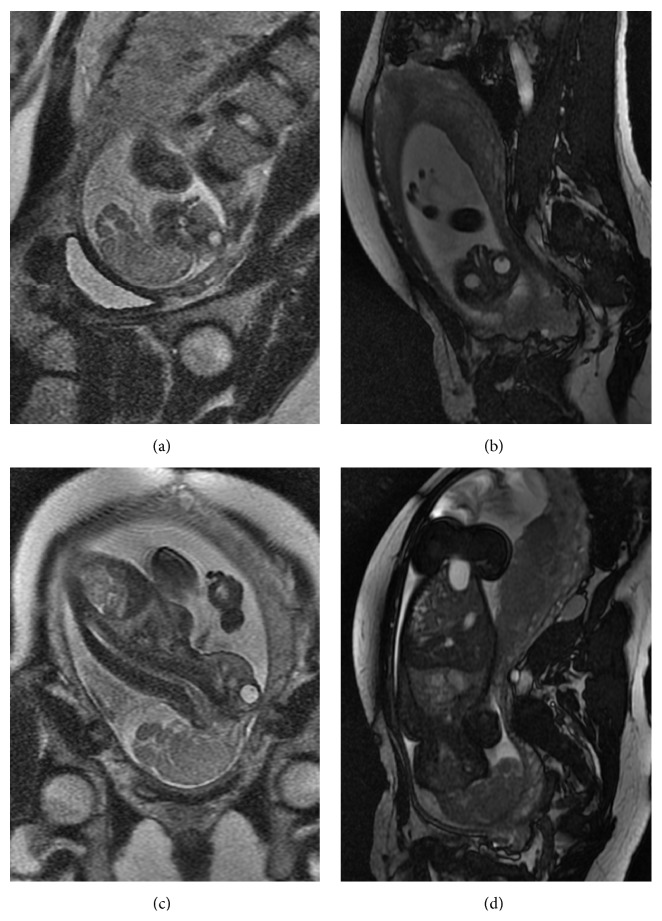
(a) Sagittal view fetal MRI demonstrating the absence of the calvarium with large mass of brain tissues hanging outside of the skull base and an incomplete layer of vascular epithelium covering the deformed brain. (b) Coronal view fetal MRI showing the intact skull base with normal appearing orbits and nasal cavity. (c) Sagittal view fetal MRI depicting the absence of the calvarium but intact orbits and skull base. The spine is normal without evidence of myelomeningocele. (d) Coronal view fetal MRI demonstrating exencephaly with grossly intact body organs.
